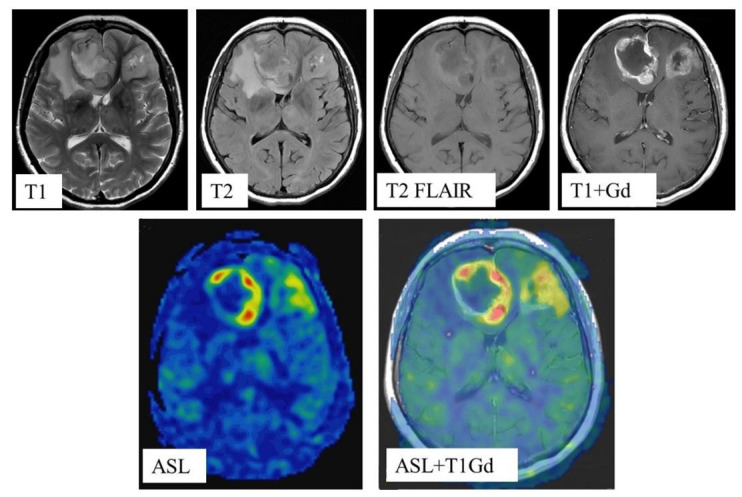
MRI scans and quantitative CBF maps of anterofrontal region IDH1-wildtype glioblastoma. ASL perfusion showed high TBF values (247.2 mL/100 g/min).
